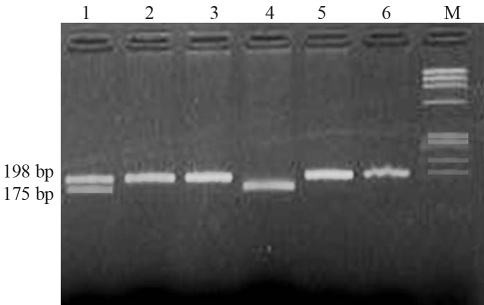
Detection of MTHFR polymorphism. Lanes 2, 3, 5 and 6 showing wild type (CC) genotype, lane 1 showing mutant heterozygous (CT) genotype and lane 4 showing mutant homozygous (TT) genotype, M showing molecular weight Marker.
